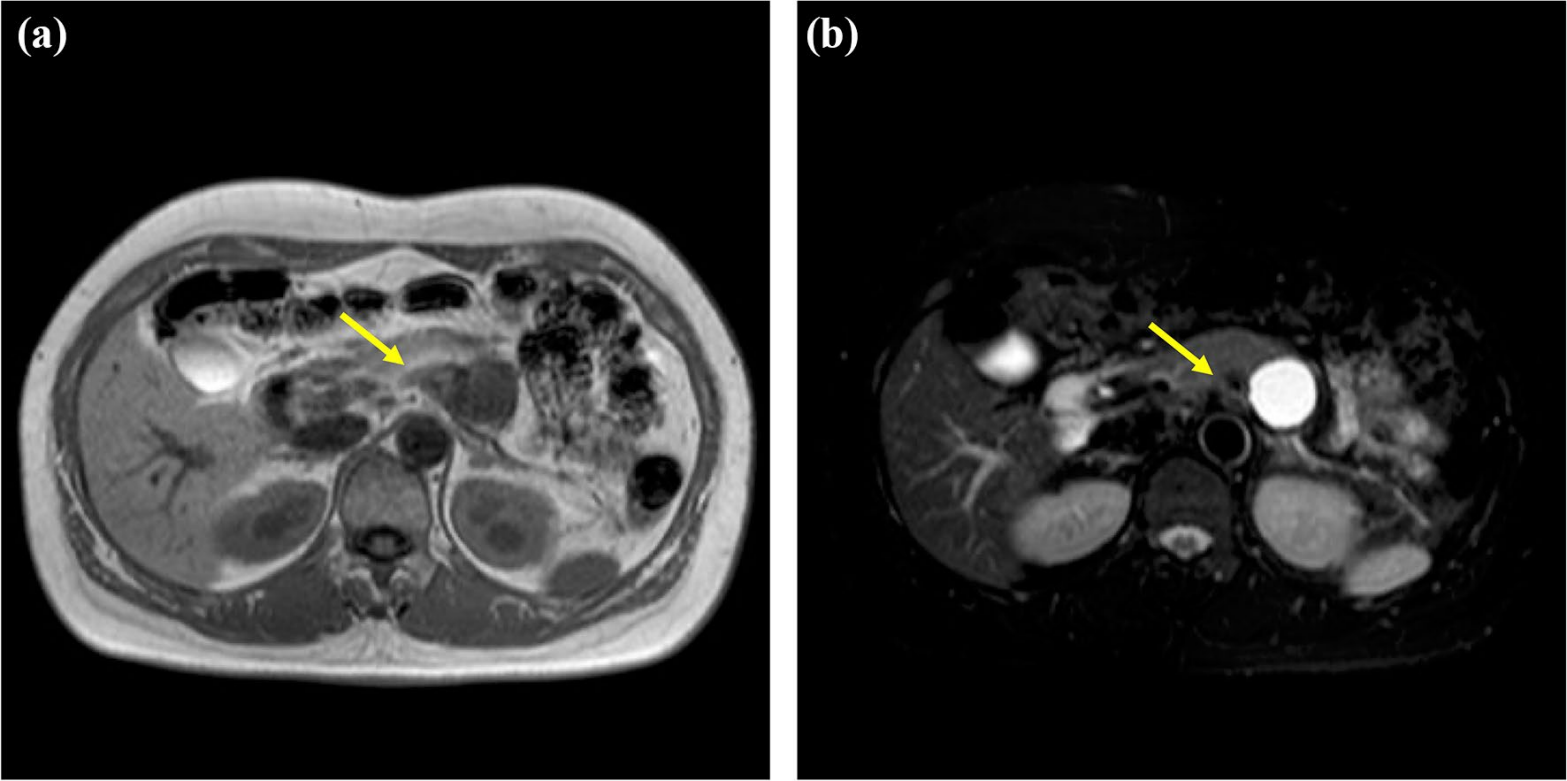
Magnetic resonance imaging showed a 3 cm-diameter unilocular cystic lesion in the pancreatic body, and a soft tissue lesion around the splenic artery (yellow arrows) that was hypointense on T1-weighted (a) and hypointense on T2-weighted images (b)
